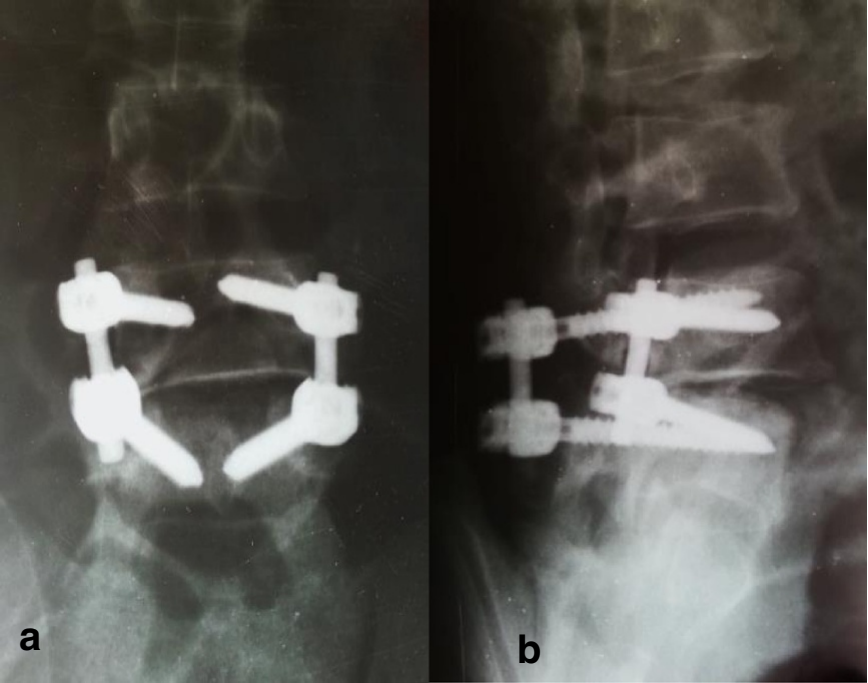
Postoperative antero-posterior (a) and lateral (b) radiographs of the lumbar spine. Good reduction is shown with short segment posterolateral fixation.
